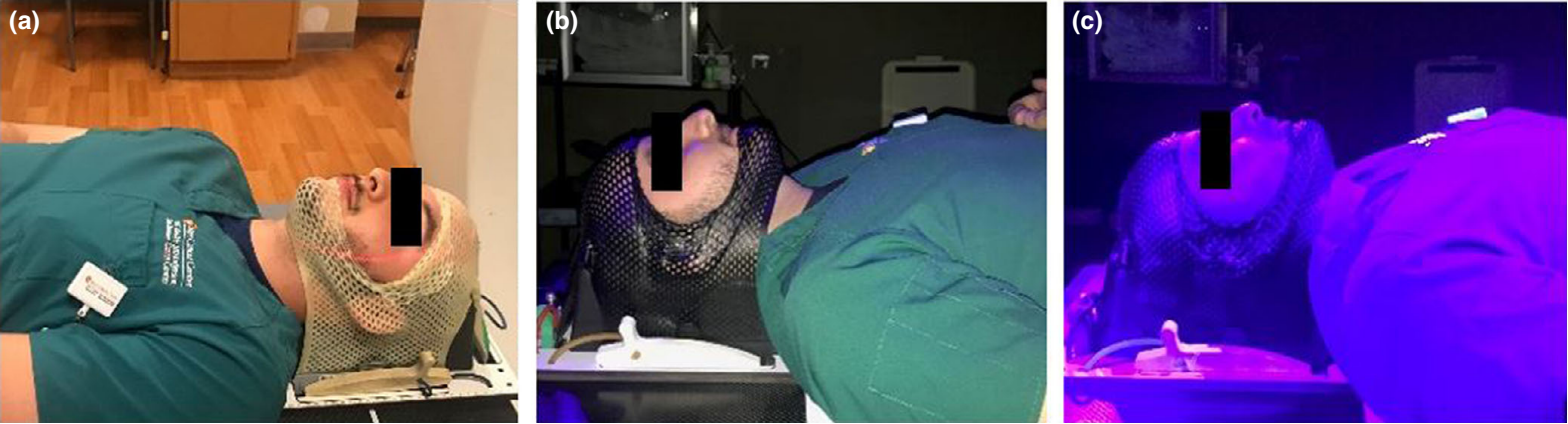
(a) Initial set up of subject in CT room shows the original color of the mask, (b) subject setup in radiation treatment room with mask spray painted matte black, and (c) subject in treatment room with lights from SGRT system (CRAD Catalyst HD).
